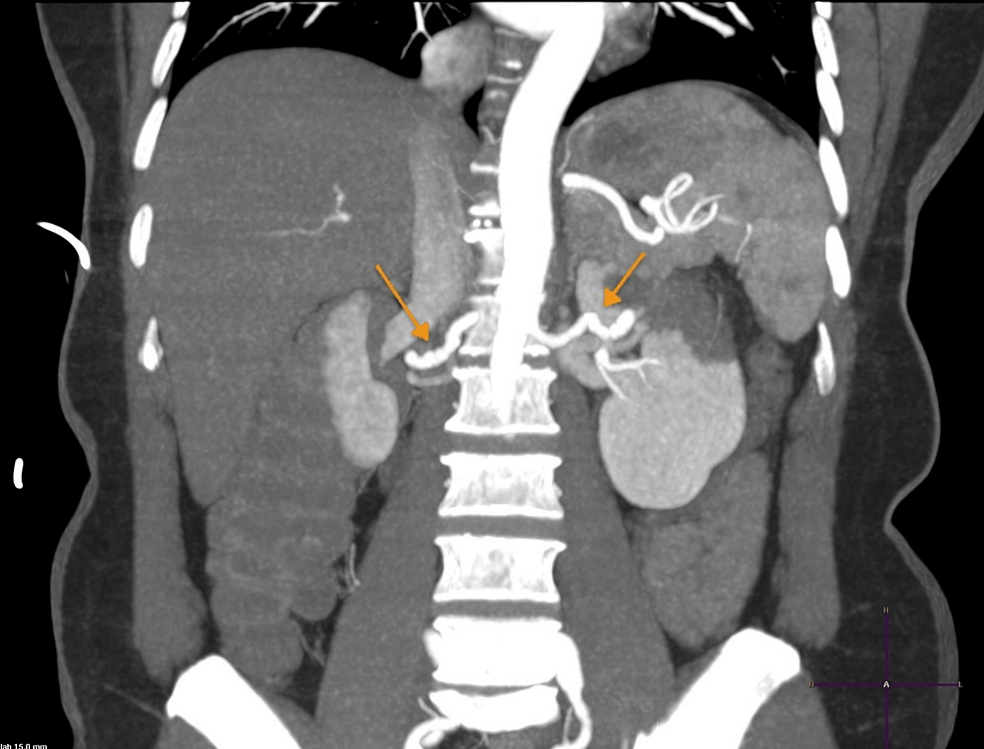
CT angiogram of the abdomen showing mild beading of the mid-right and left renal arteries and a tortuous left renal artery.
